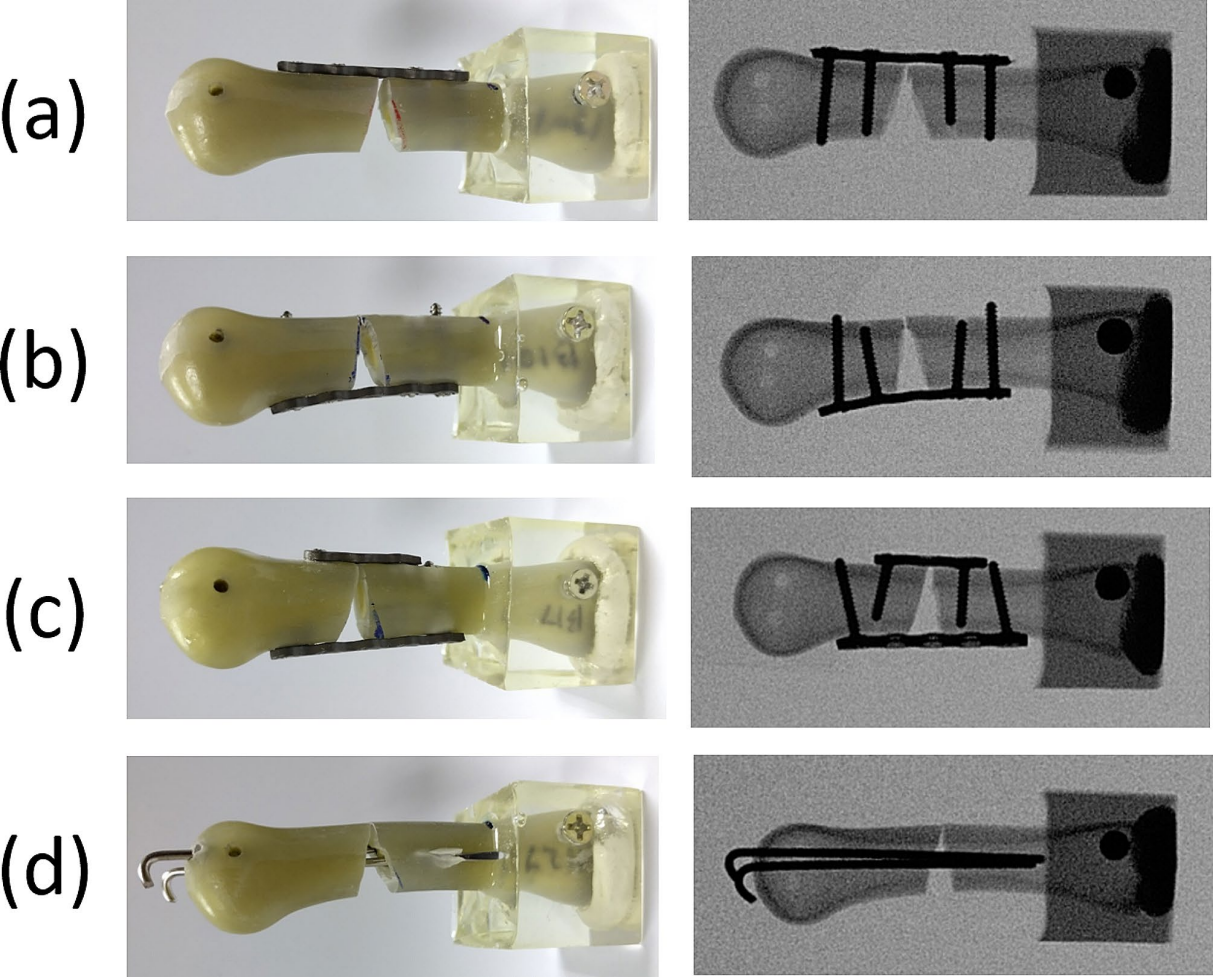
Photographs (left) and radiographs (right) of four types of fixation methods involving the use of (a) a locked plate with four locked bicortical screws on the dorsal side, (b) a locked plate with four locked bicortical screws on the volar side, (c) a combination of a 3-hole locked plate with two locked bicortical screws on the dorsal side and a 5-hole locked plate with two locked bicortical screws on the volar side, and (d) two K-wires in a cross-pin fixation configuration
